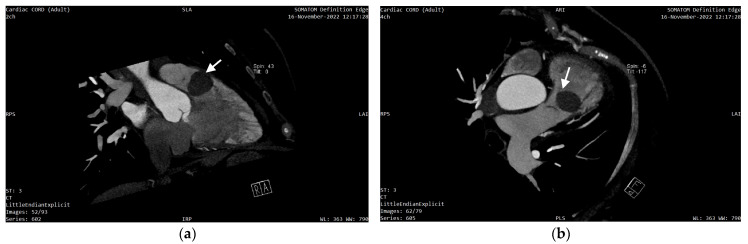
Cardiac CT reconstruction showing tumor (white arrow) correspondence to the neighboring anatomical structures: (a) two chambers reconstruction; (b) four chambers reconstruction.
